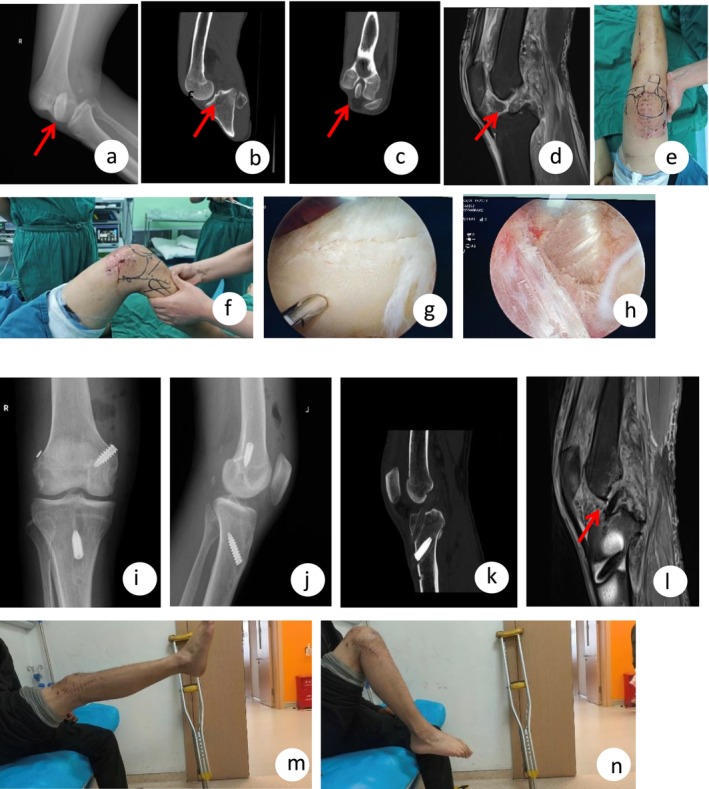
Case 1—Imaging and intraoperative findings of a case with right knee rotatory dislocation. (a) Anteroposterior radiograph at admission demonstrating rotatory dislocation of the right knee. (b, c) Three‐dimensional CT reconstructions on admission confirming knee dislocation with the patella displaced and incarcerated within the femoral intercondylar notch. (d) Post‐reduction MRI following emergency open reduction, revealing ruptures of the anterior and posterior cruciate ligaments. (e–h) Preoperative examination under anesthesia and intraoperative arthroscopic views during multiligament reconstruction performed two weeks after admission. (i–l) Postoperative anteroposterior and lateral radiographs along with CT images of the right knee demonstrating satisfactory positioning of suture anchors and reconstructed ligament tunnels. (h) MRI of the reconstructed anterior and posterior cruciate ligaments following multiligament reconstruction, showing restored ligament integrity. (m, n) Follow‐up photographs at one month postoperatively, showing active knee extension and flexion achieved by the patient.
